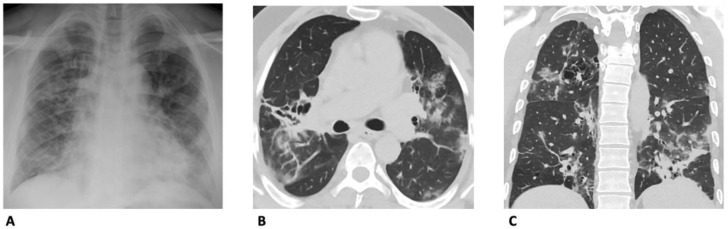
Comparison between chest X-ray and CT findings suggestive of SARS-CoV-2-related pneumonia in a 37-year-old man. (A) Chest X-ray shows the presence of multiple confluent mixed alveolar and linear opacities. CT images in the axial (B), and in the coronal plane (C) with window width and level for the evaluation of lung parenchyma allows to correctly identify the presence of the confluent ground-glass opacities, consolidations, and the additional finding of emphysema associated with bronchiectasis in the right upper lobe and both lower lobes.
